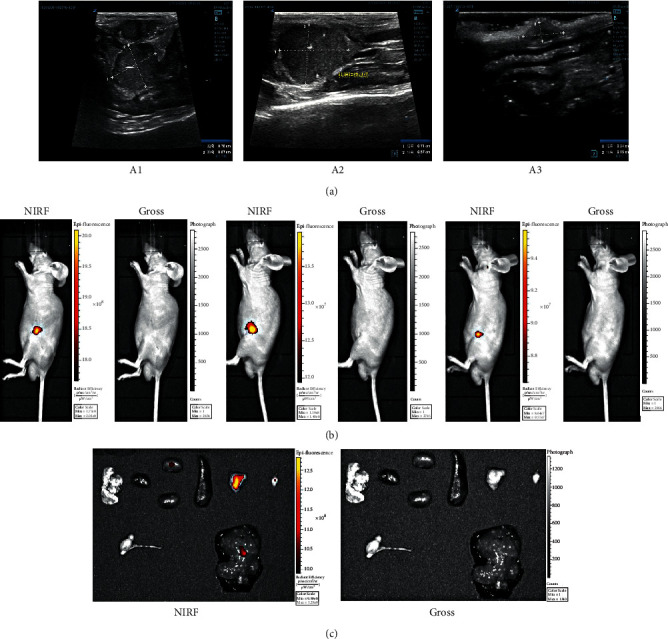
Imaging and targeting pancreatic orthotopic tumor of group A. (a) B-mode ultrasound imaging of tumors; (b) NIRF imaging of tumors; and (c) NIRF imaging of organs. The lungs, heart, kidney, spleen, tumor *in situ*, and inguinal lymph node are from left to right in the first row. The testes and spermaduct, and liver are in the second row respectively.
